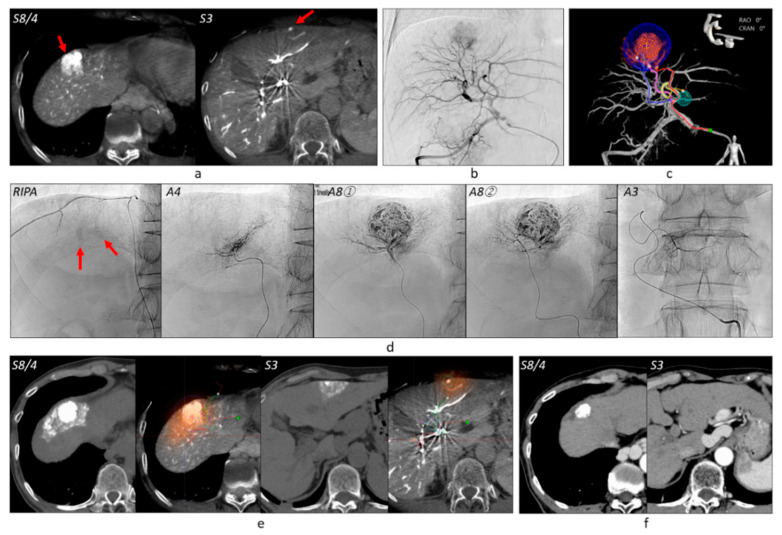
Virtual parenchymal perfusion functionality. (a) The first-phase CBCTHA depicted two HCCs of 28 mm and 7 mm in diameter, respectively (arrows). (b) The tumor in S8/4 was depicted on a common hepatic arteriogram, but a small HCC in S3\ could not be depicted. (c) AFD could identify three tumor feeders for the tumor in S8/4 and one feeder for the tumor in S3. (d) First, the anterior branch of the right IPA was embolized because the tumor was protruding into the diaphragm from the liver surface. During TACE of this branch, iodized oil accumulation in the tumor was confirmed on a spot radiograph (arrows). Then, one branch of A4 and two branches of A8 were subsequently embolized and Grade 2 portal vein visualization was obtained. Finally, one branch of A3 was embolized, but the portal veins were not opacified (Grade 0). Selective DSA of each vessel was not performed. (e) The virtual embolized areas and the real embolized areas on CT performed 1 week after TACE were well correlated. (f) The tumor in S8/4 has remained well controlled and the tumor in S3 has disappeared 4 years and 1 month after TACE. Abbreviations: S8/4, boundary between segments 8 and 4; S3, segment 3; RIPA, right inferior phrenic artery; A4, medial subsegmental artery of the left hepatic artery; A8, anterior superior subsegmental artery of the right hepatic artery; A3, superior lateral subsegmental artery of the left hepatic artery.
